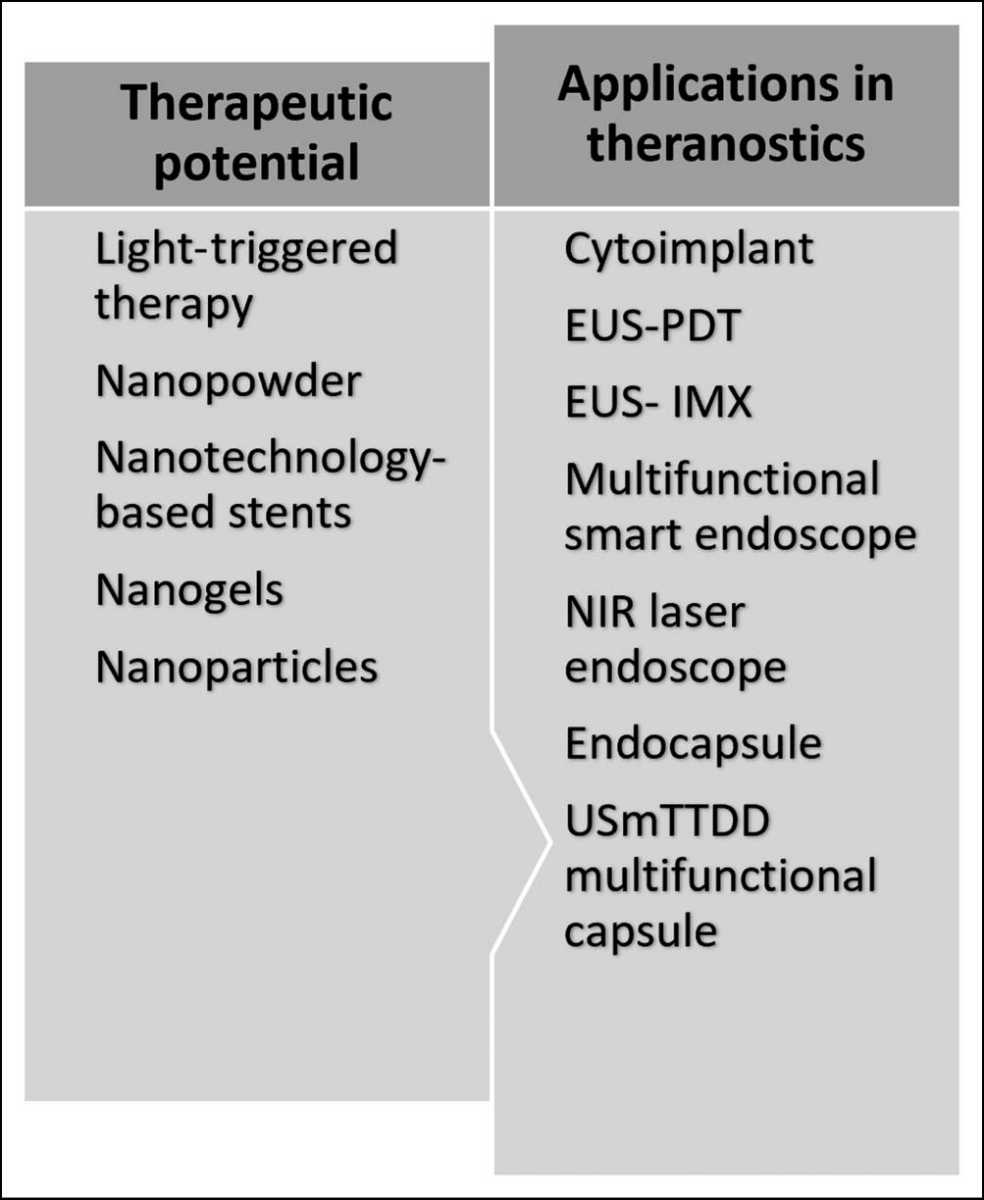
Summary of the described clinical and experimental techniques. EUS-PDT, endoscopic ultrasound-photodynamic therapy; EUS-IMX, endoscopic ultrasound-immunotherapy; NIR, near-infrared; USmTDD, ultrasound-mediated targeted drug delivery.
